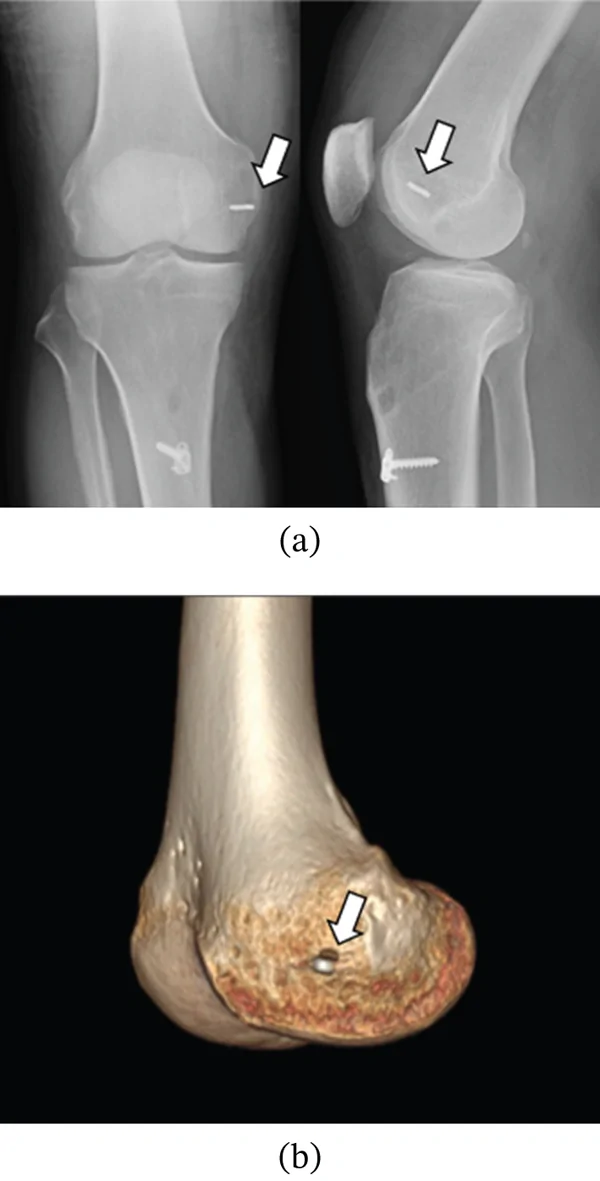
(a) Radiograph of the right knee seven (7) days after surgery showing that the EndoButton turned in a different direction and moved into the femoral tunnel (white arrow). (b) Three‐dimensional computed tomographic image demonstrating migration of the EndoButton into the femoral tunnel, with horizontal displacement of the button and a superior cortical defect/enlargement around the femoral tunnel outlet.
